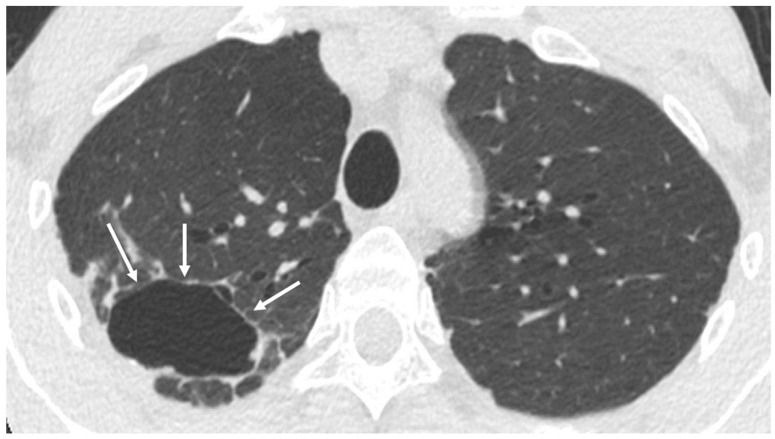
Incidental finding of right upper lobe (RUL) cavitary lesion in low-dose computed tomograph (LDCT). Axial LDCT of 41-year-old male demonstrates cavitary lesion measuring 5.4 cm in RUL (arrows). Further workup is recommended to determine etiology.
